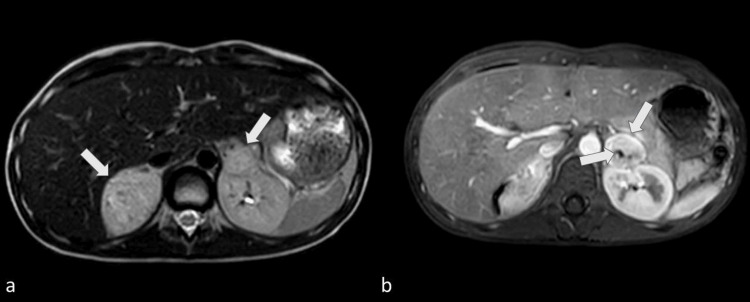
Abdominal MRI demonstrated bilateral adrenal masses, measuring 5.4 × 4.0 × 5.0 cm (right) and 3.0 × 2.5 × 3.3 cm (left), with no evidence of local invasion or lymphadenopathy. a: T2-weighted turbo spin-echo images show bilateral hyperintense adrenal lesions (white arrows); b: Gadolinium-enhanced T1-weighted images reveal early heterogeneous enhancement and areas of cystic degeneration (white arrows), suggestive of bilateral pheochromocytomas
